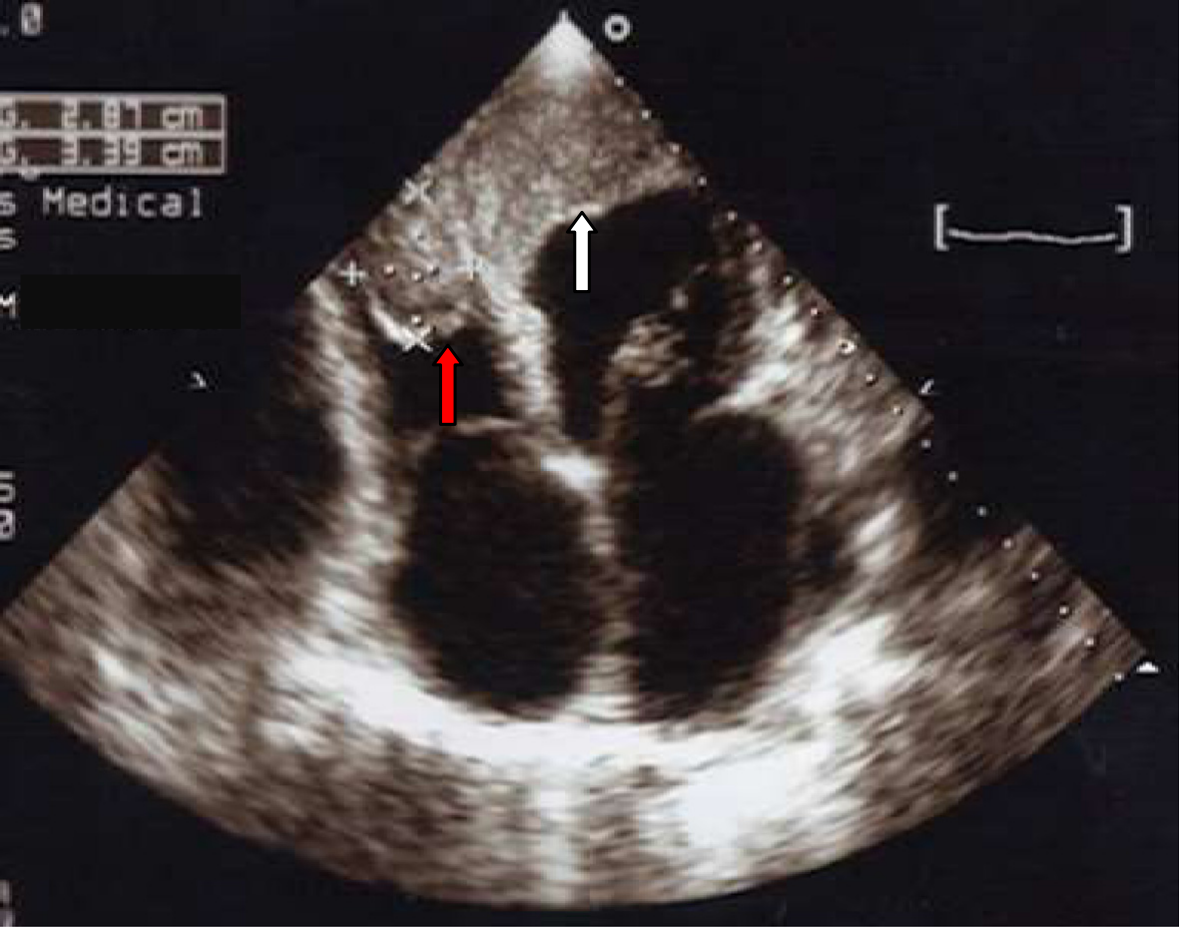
Apical four chamber view showing right ventricular thrombus (red arrow) and left ventricular thrombus (white arrow). The left ventricular thrombus is larger than the right.
